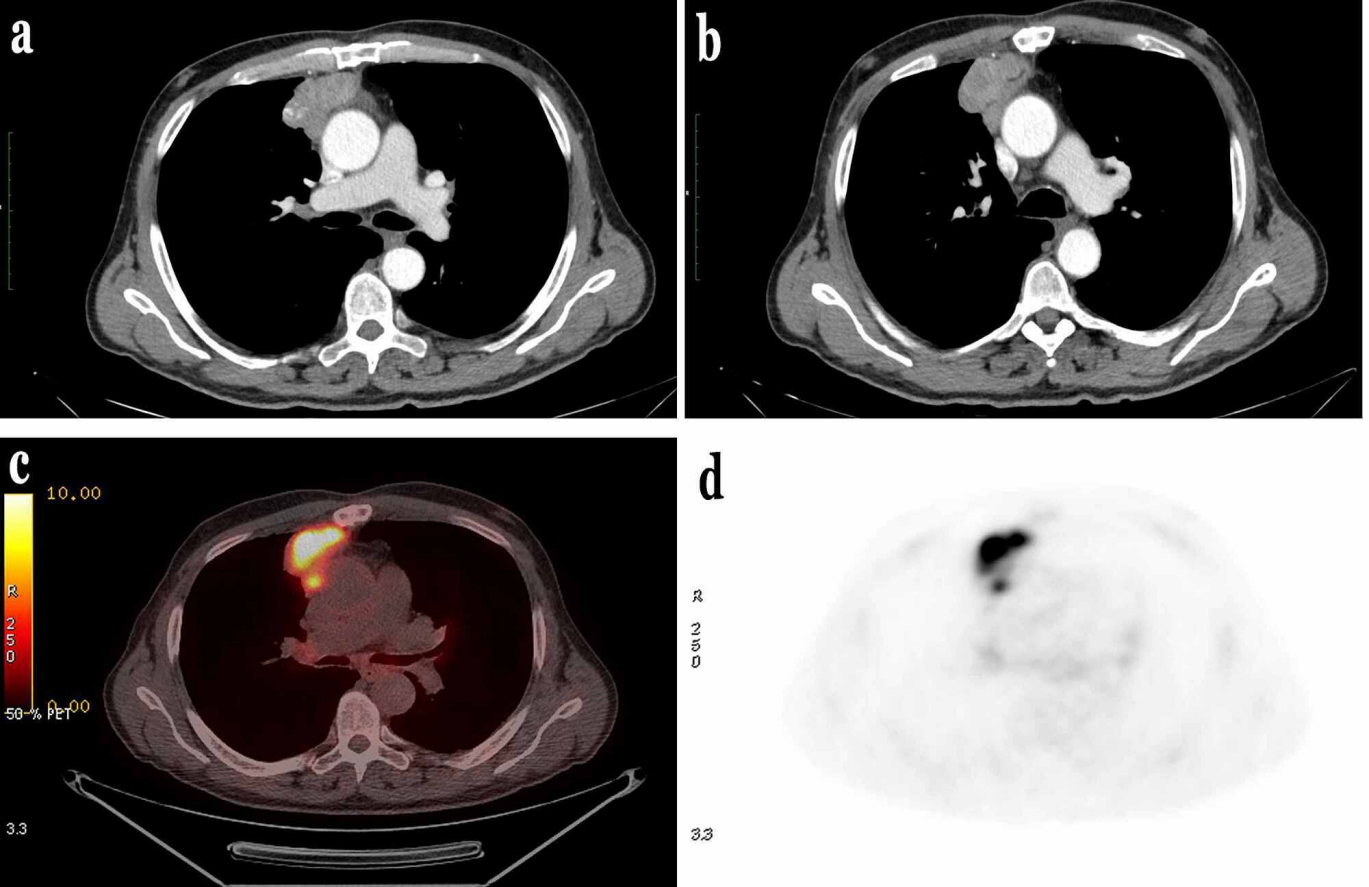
Imaging findings. (a,b) Chest CT images demonstrate a tumor mass in the anterior upper mediastinum.  (c,d) FDG-PET scan images demonstrate high uptake in the mediastinal mass without metastasis.
